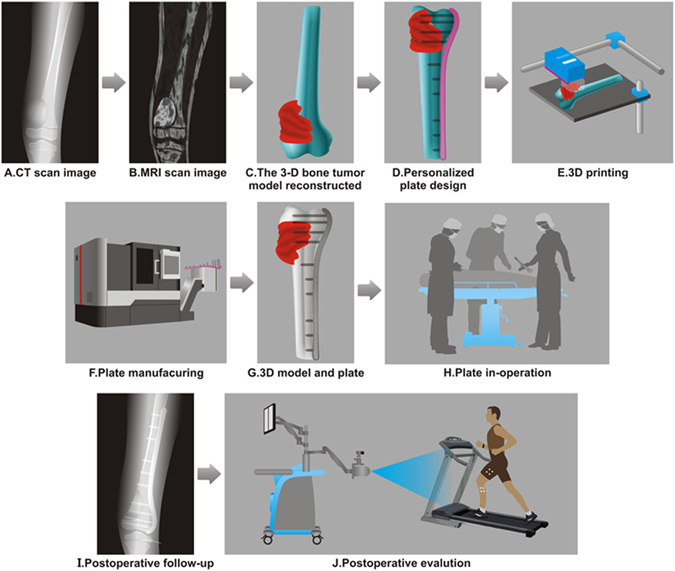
The current global procedure for the designed clinical study. (A) CT scan is first performed on the tumorous bone. (B) The MRI scan image is then performed on the tumorous bone. (C) In accordance with the merged CT-MR image, a 3D model of the tumorous bone is constructed using Mimics software, with tumor being highlighted in red. (D) The 3D model shown in C is then used to design a personalized titanium plate (pink) using UG software. (E) 3D printing is used to print the bone model shown in (C). (F) A numerical control machine is used to fabricate the personalized titanium plate shown in (D). (G) The fabricated titanium plate is combined with the 3D printed tumorous bone to match the model shown in (D) in order to test if the plate matches the tumorous bone as modeled. (H) The fabricated personalized plate is then used to fix the bone structure after the surgery removal of osteosarcoma and the bone repair using the mixture of allograft bone and cement. (I) The recovery of the patient is followed by X-ray examination and gait analysis.
